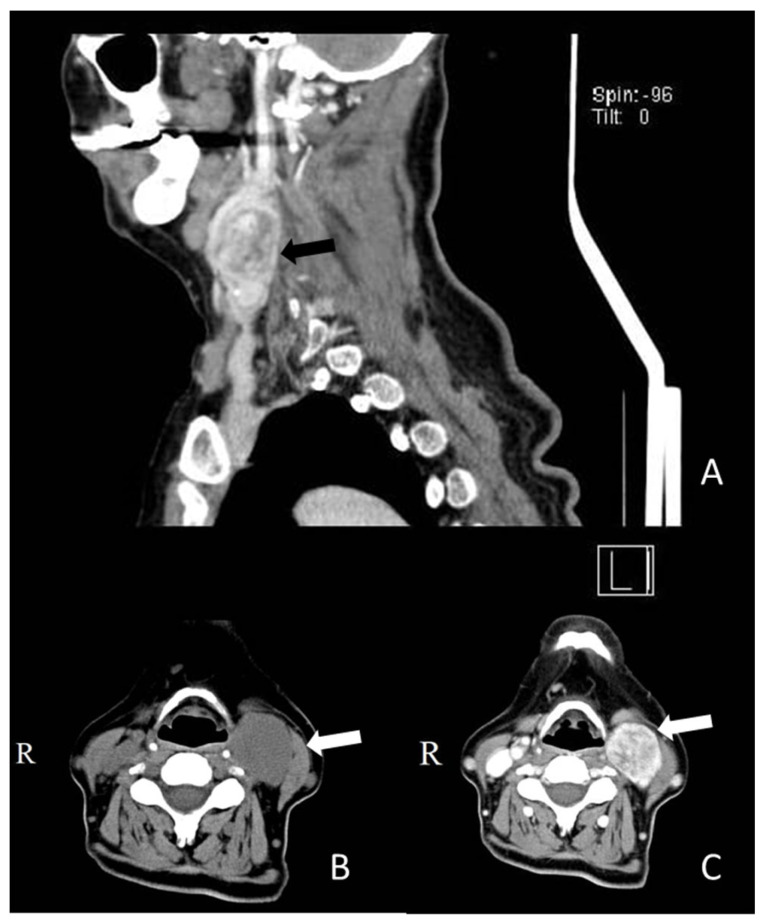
(A) CT scan of the left cervical region (sagittal section); the black arrow is pointing towards the tumoral mass. (B) CT scan of the left cervical region without contrast agent (transversal section); the white arrow is pointing towards the tumoral mass. (C) CT scan of the left cervical region with contrast agent (transversal section); the white arrow is pointing towards the tumoral mass.
